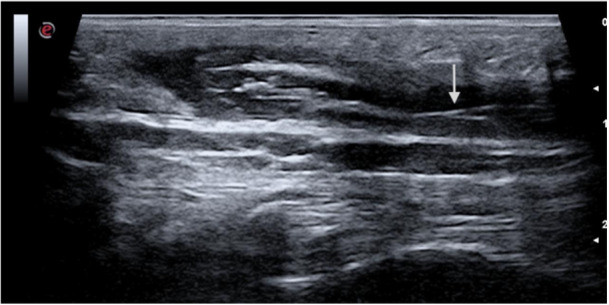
High frequency ultrasound of the natal cleft showing a collection and hair follicle in the sinus (arrow).
